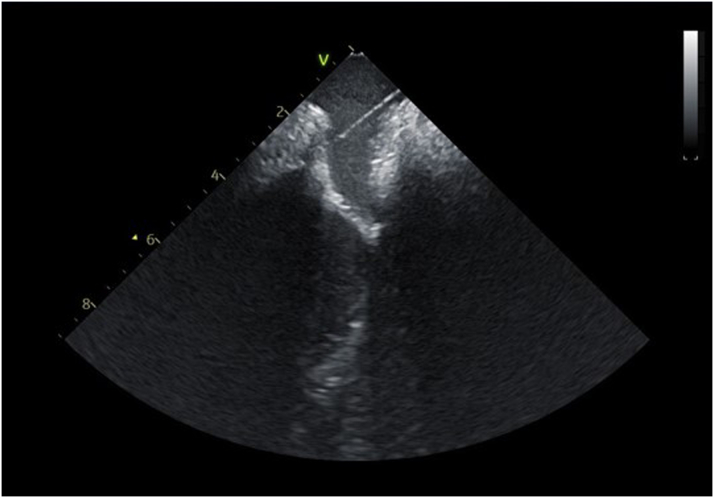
Intracardiac Echocardiography of Transpulmonary Puncture Intracardiac echocardiography image of trans-septal needle extending from the superior vena cava (rightward) into the floor of the proximal left pulmonary artery directed toward the epicardial space.
